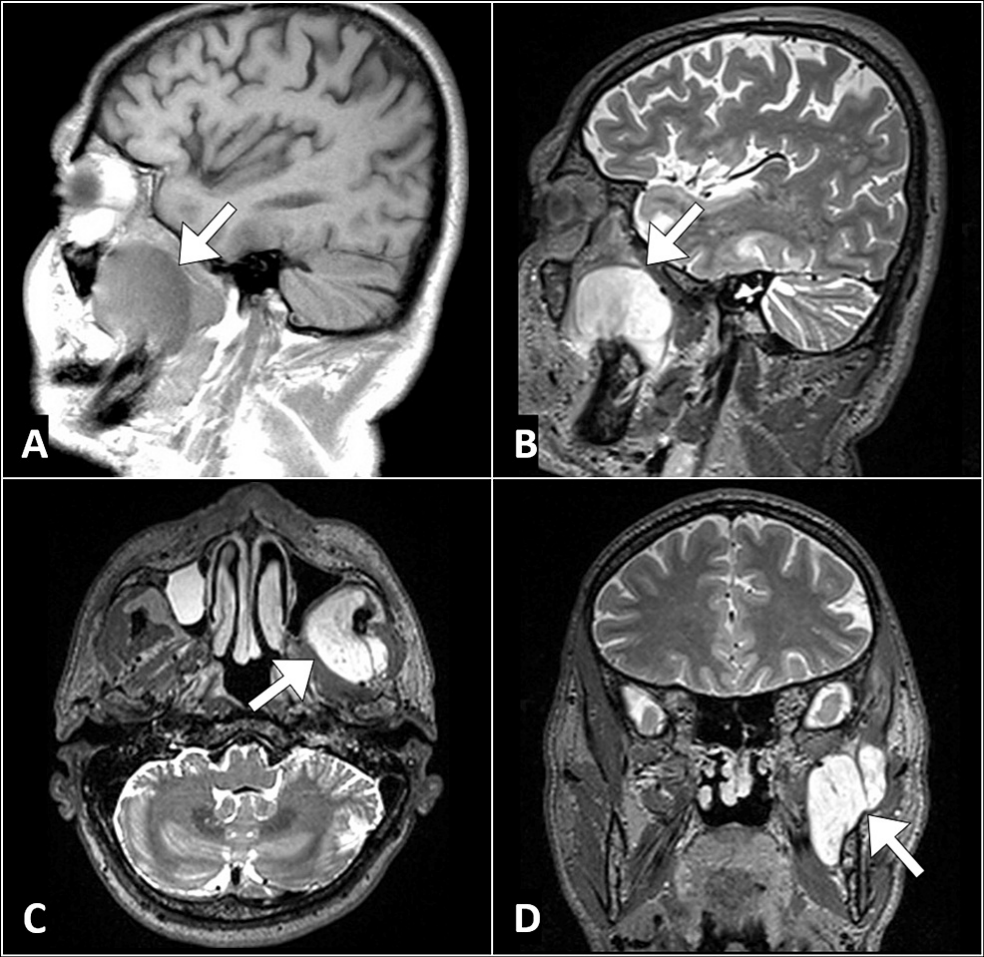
Magnetic resonance images of the brain reveal a lesion in the masseteric muscle (arrow) characterized by low signal intensity on the T1-weighted image (A) and high signal intensity on the T2-weighted image (B). Similar lesions with corresponding intensities were observed in the pterygoid muscles (arrow) as depicted in the axial (C) and coronal (D) T2-weighted images.
